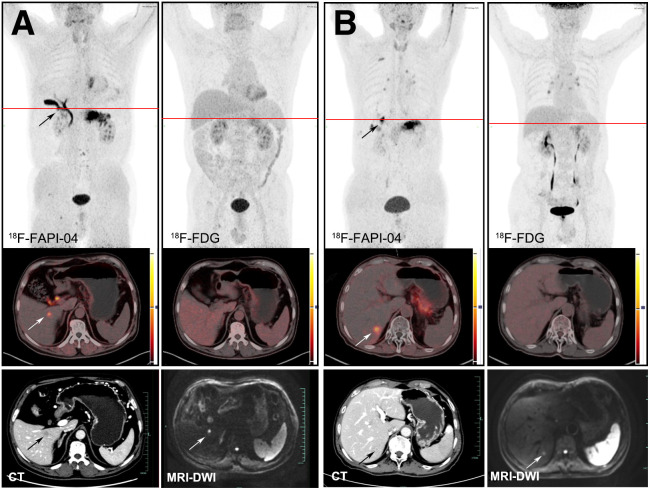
Typical PET (top), PET/CT (middle), and CT and MR (bottom) images of hepatic metastases obtained using 2 tracers in 2 patients (A and B). Lesion is marked by arrows. DWI = diffusion-weighted imaging.
